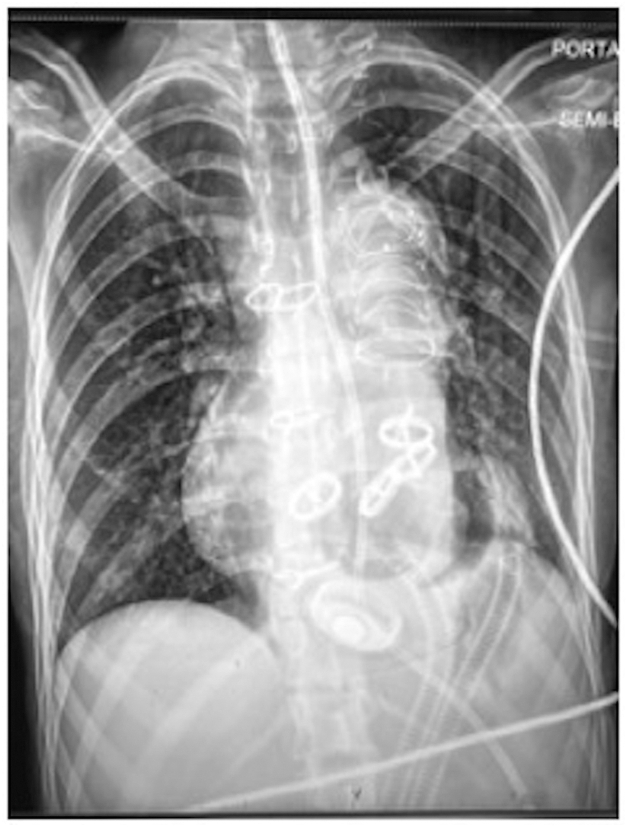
Chest radiograph showing temporary total artificial heart and frozen elephant trunk with distal aspect in the descending aorta.
